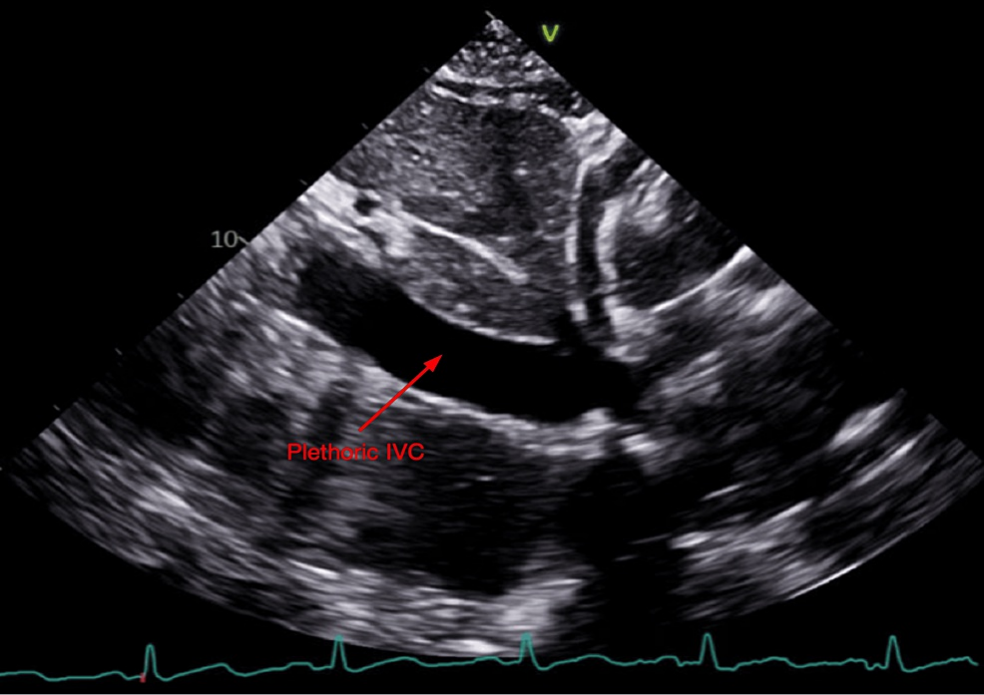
Subcostal IVC echocardiogram - Plethoric IVC IVC, inferior vena cava.
